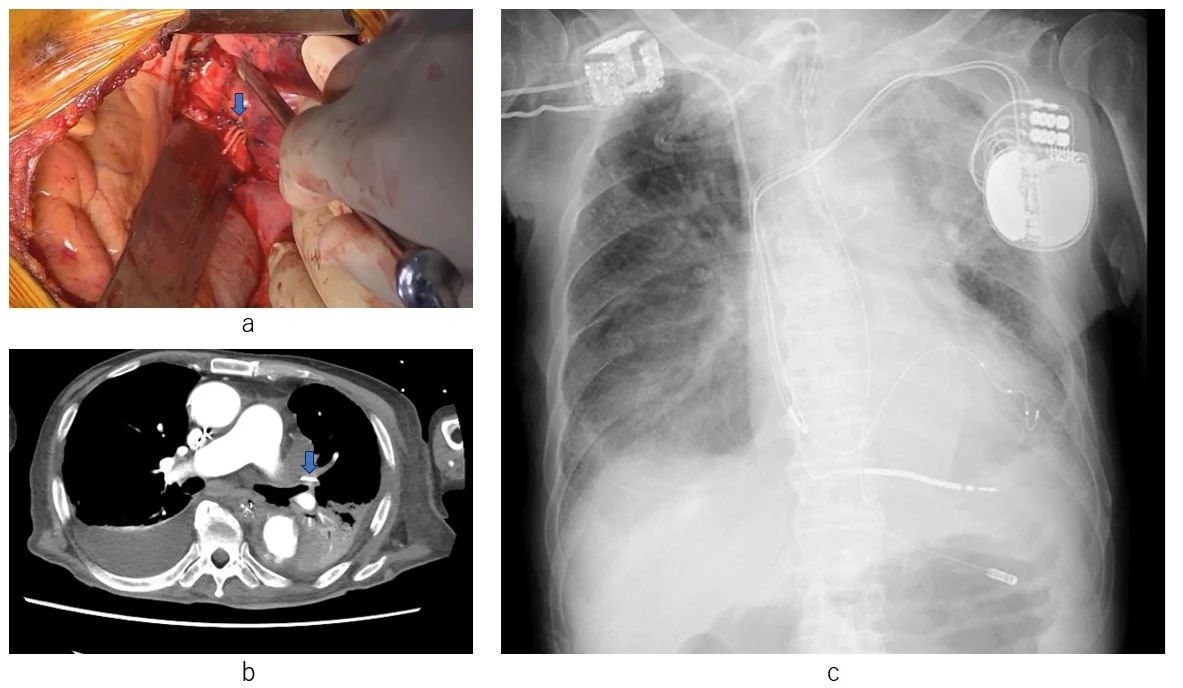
Bronchial ligation and postoperative findings. (a) Intraoperative photograph showing ligation of the left upper lobe bronchus under thoracoscopic guidance. The blue arrow indicates a hemoclip placed to reinforce the distal knot of the ligature. (b) Postoperative contrast-enhanced computed tomography showing a hemoclip marking the site of the ligated left upper lobe bronchus. The arrow indicates the hemoclip. (c) Chest radiograph after bronchial ligation showing good expansion of the left lung, except for residual atelectasis of the left upper lobe.
